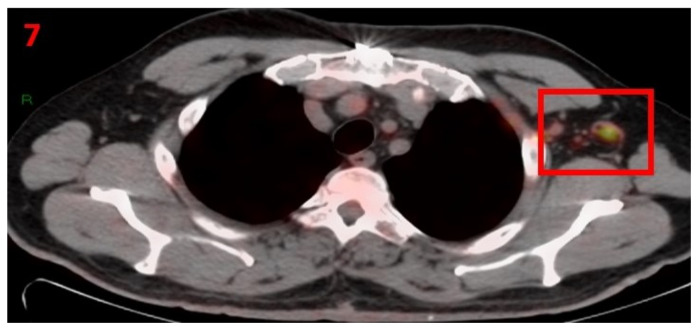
[18F]FDG PET-CT showing hypermetabolic uptake of in 7th patient with lymphadenopathy in the left axillary region (red square).
